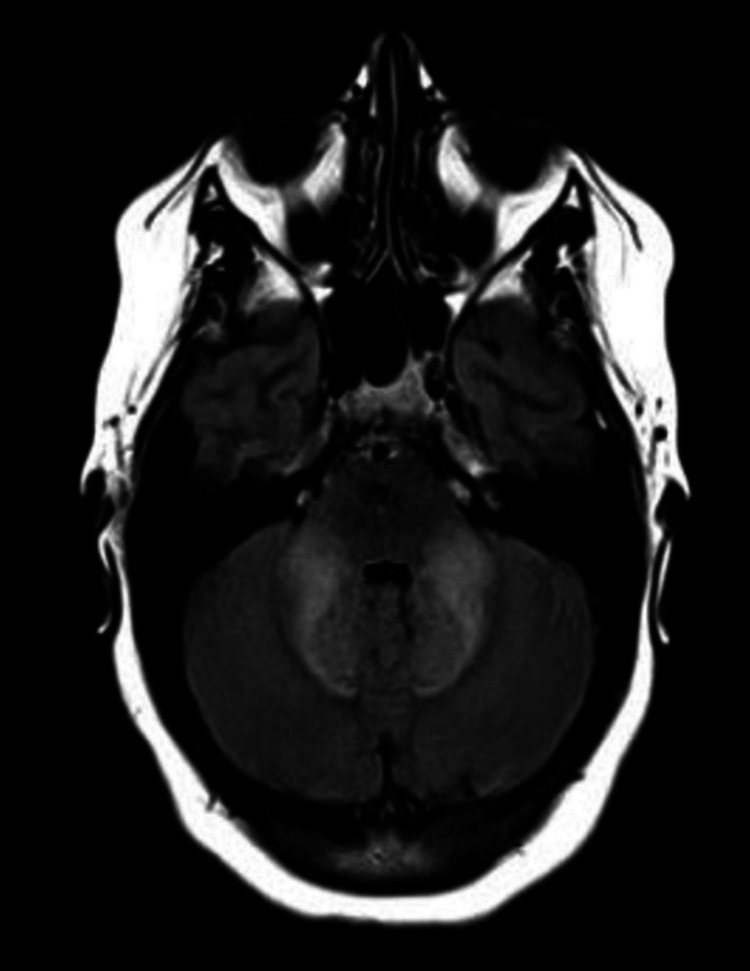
FLAIR image reflecting the symmetry and extent of the hyperintensities in the brachium pontis FLAIR: fluid-attenuated inversion recovery
